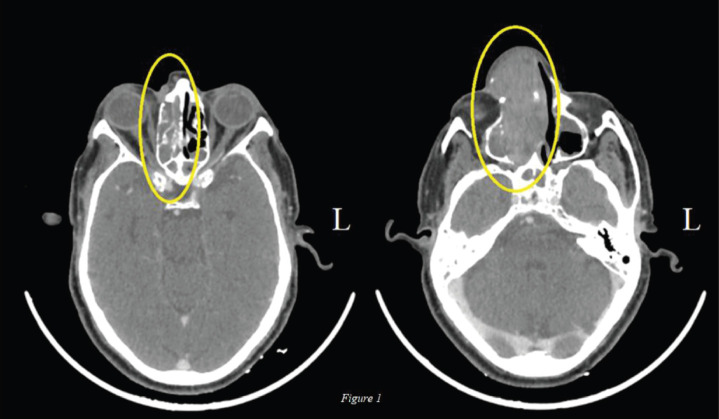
Axial view: Pre-OMCT CT of nasal cavity and sinuses: Interval increase in the mass (9.7 × 4.6 cm) involving right nasal cavity lesion creeping through nasopharynx to skull base without bony erosion, also inferomedially in right orbit and further in right paranasal sinuses.
